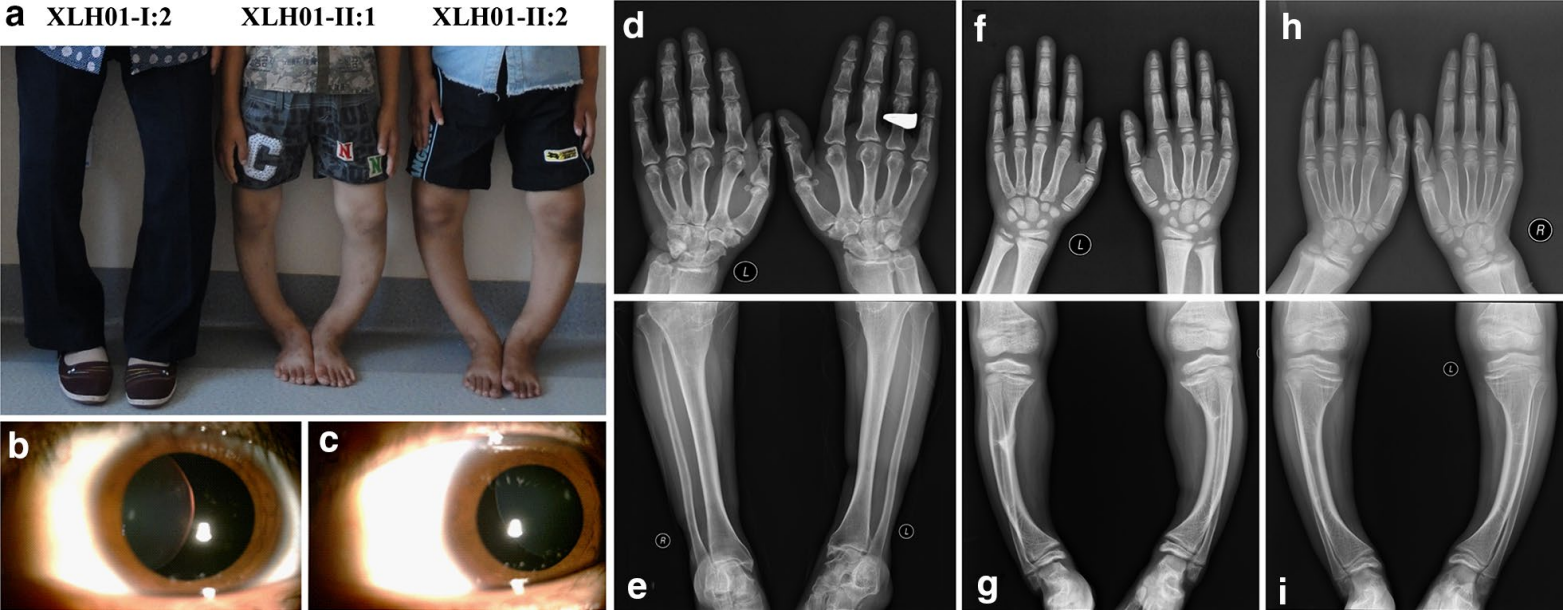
Clinical presentation for family XLH01. a Medial bowing in the patients XLH01-I:2, XLH01-II:1 and XLH01-II:2. b, c Anterior segment photography indicates dislocation of lens toward nasal superior side in both eyes. d–i Radiographic findings reveal dolichostenomelia in the digits of patient XLH01-II:2 (h), but not in patient XLH01-I:2 (d) or XLH01-II:1 (f). Widening of both proximal and distal tibial metaphysis in patients XLH01-II:1 (f, g) and XLH01-II:2 (h, i) are shown. Medial bowing is found in all three patients (e, g, i).
